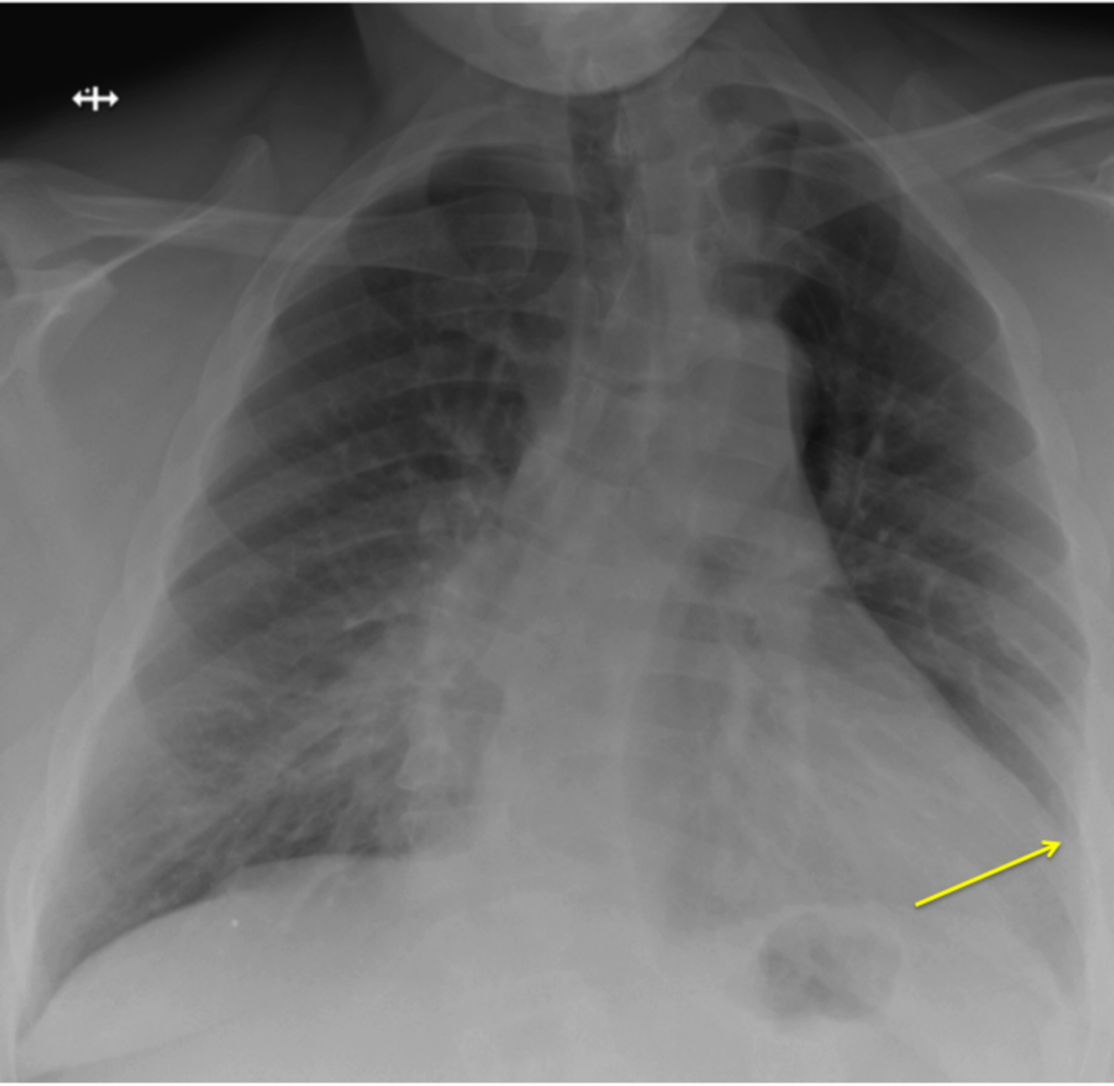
Chest X-ray on admission revealed normal lung findings and cardiomegaly. Yellow arrow indicating left lateral boarder of enlarged cardiac silhouette.
